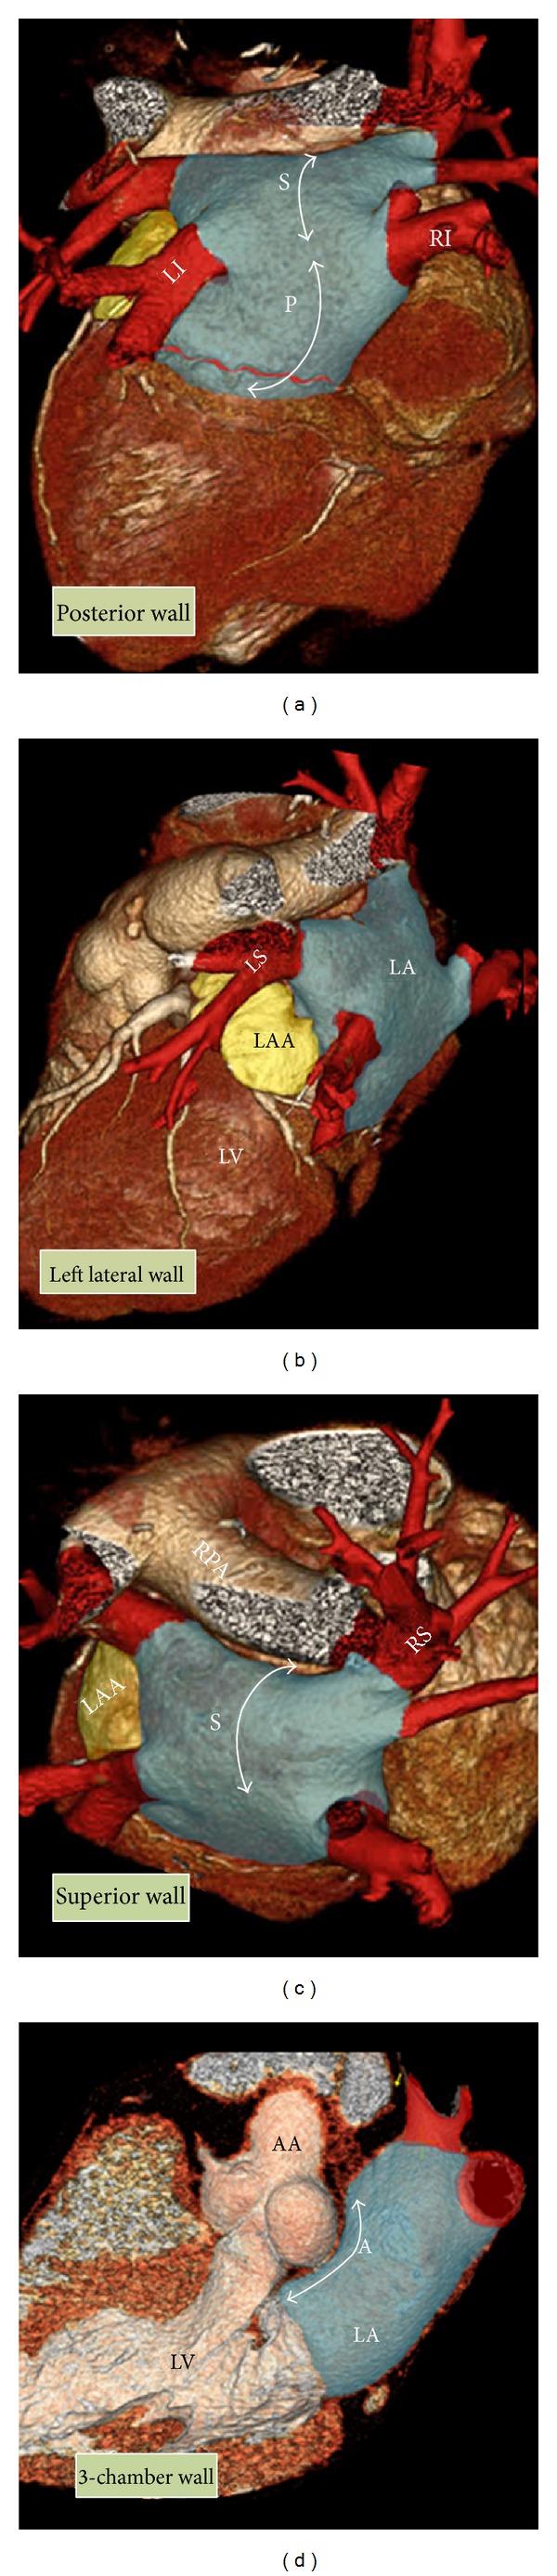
Anatomic relationship of the left atrium (LA). Posterior, right lateral, superior, and three-chamber view of volume rendered CT angiographies are shown. The LA (in blue) is located superiorly and posteriorly to the heart. The walls of LA include the anterior, superior, left lateral, septal, and posterior. Its superior (S) and posterior (P) walls are shown by double-headed arrows. Note close relation of the anterior (A) wall to the ascending aorta (AA). The left atrial appendage (LAA) is shown in yellow and the pulmonary veins are shown in red. The four pulmonary veins include LS = left superior, LI = left inferior, RS = right superior, RI = right inferior, LV = left ventricle.
